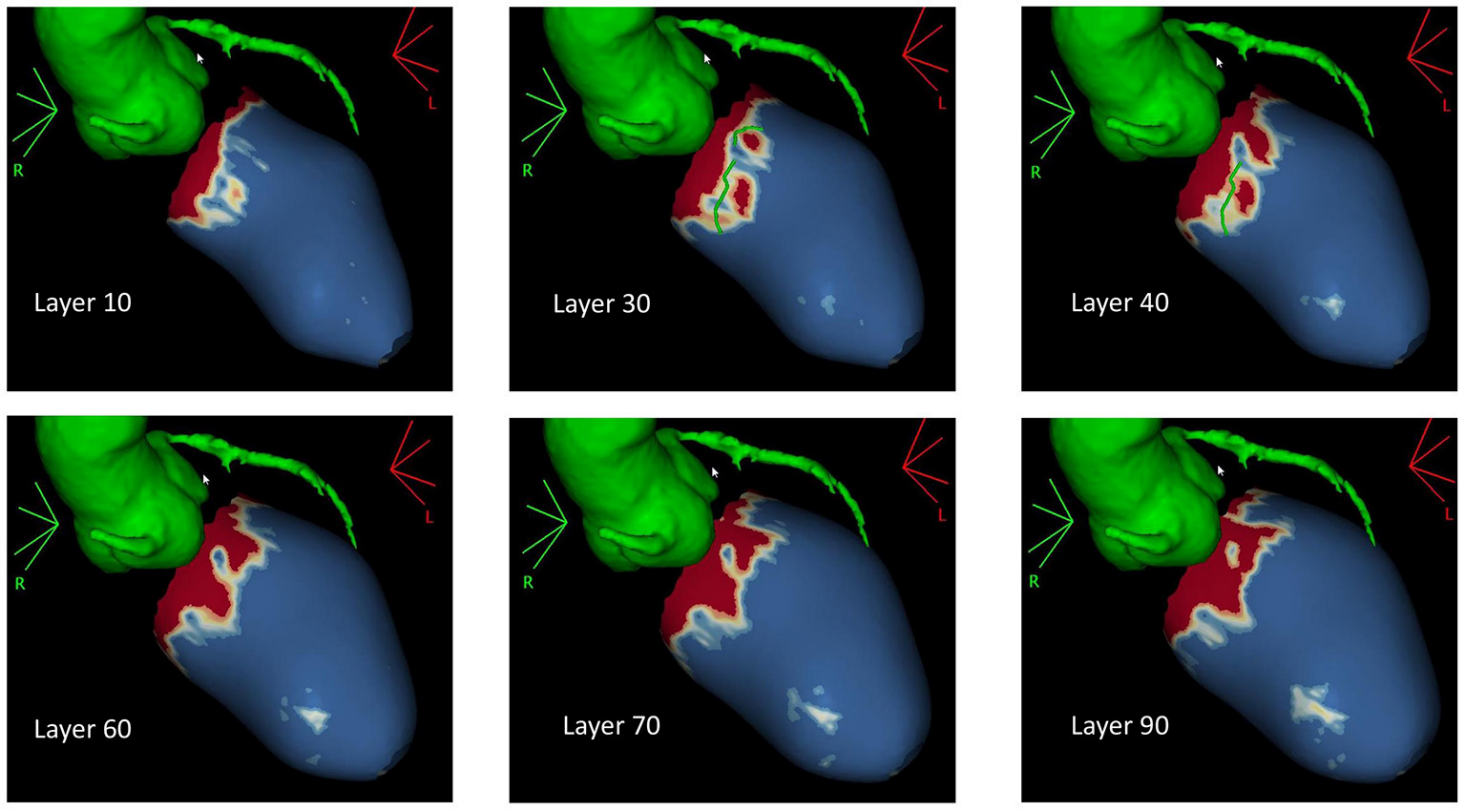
Pixel signal intensity maps obtained through post-processing of three-dimensional (3D) late gadolinium enhancement (LGE) MRI images at different layers of left ventricular (LV) wall thickness as obtained with ADAS3D software (ADAS3D Medical S.L., Barcelona, Spain). Conduction channels are evident on the mid-myocardial layers (30–40%) and are marked by green lines. Dense scar is depicted in red, while normal myocardium is represented in blue. Border zone tissue is shown in shades of red. Courtesy of Dr. Giulio Zucchelli, Second Division of Cardiovascular Diseases, Cardio-Thoracic and Vascular Department, University Hospital of Pisa, Pisa, IT, Italy.
